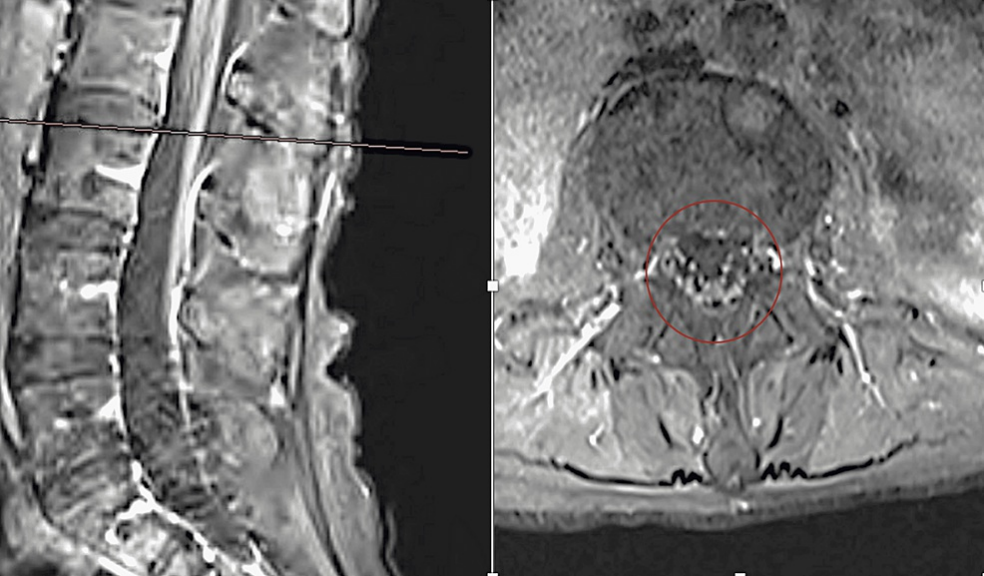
T1W contrast MRI lumbar spine with the enhancement of a thickened cauda equina and thickened enhanced conus medullaris. MRI of the patient courtesy of Dr. Marc Swerdloff, August 2021.
